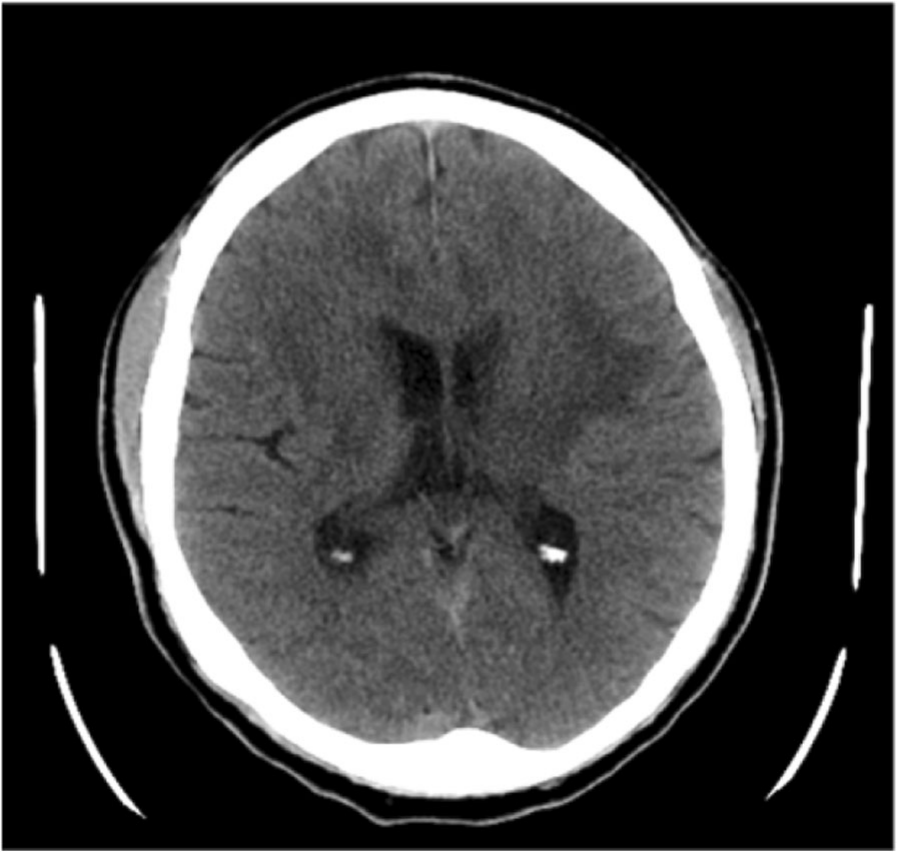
Computed tomography shows hypodense lesion within the left white matter and left basal ganglia
